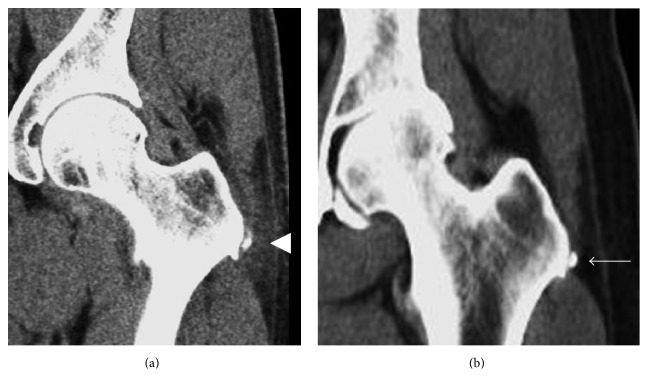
CT appearance of calcific tendinitis. (a) Acute symptomatic phase mirrors radiographic findings with ill-defined, amorphous calcification and associated soft tissue edema (arrowhead). (b) Calcification becomes better defined with resolution of soft tissue edema as the calcific tendinopathy progresses to the chronic phase (arrow).
